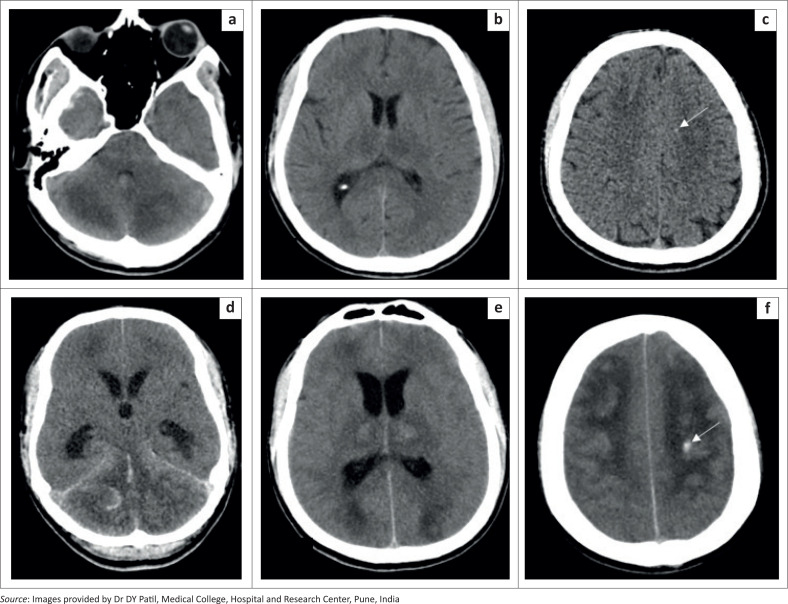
[Case 1]: Axial CT section in a 25-year-old male demonstrating ill-defined symmetric hypodense areas involving the bilateral thalami, cerebellar white matter and bilateral frontal subcortical and deep white matter (a–c). Tiny hyperdense foci were seen within some of these regions (white arrow). A repeat CT study performed a day later revealed an increase in the hypodensities and the haemorrhagic foci with intraventricular extension of the haemorrhage and resultant obstructive hydrocephalus (d–f).
